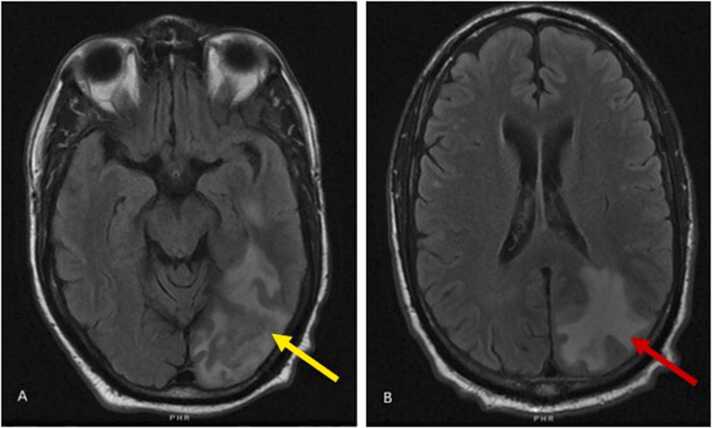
MRI brain T2 flair images – inferolateral left temporal lobe lesion with surrounding vasogenic edema (yellow arrow) and 2.7 cm occipital lesion (red arrow).
